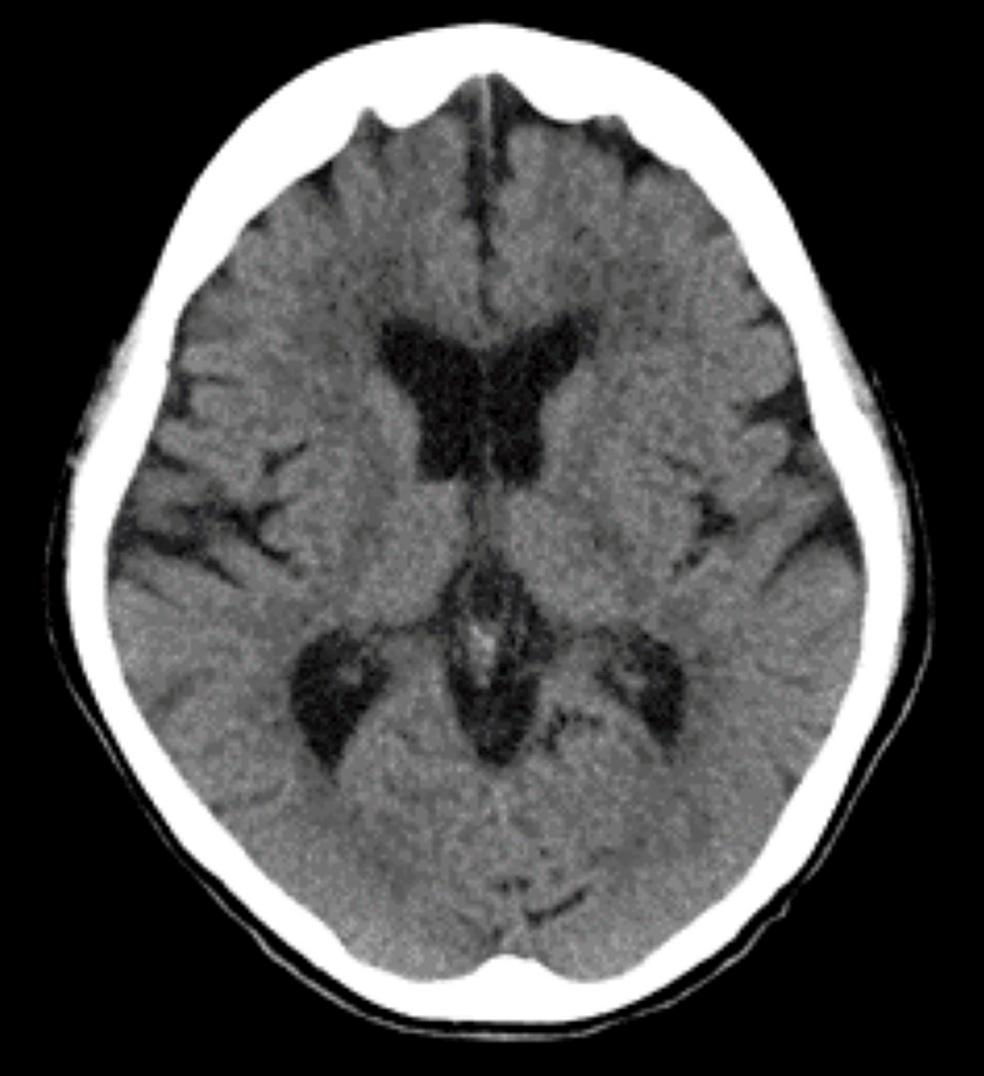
Head computed tomography on admission reveals no intracranial lesions or diseases causing the altered level of consciousness.
